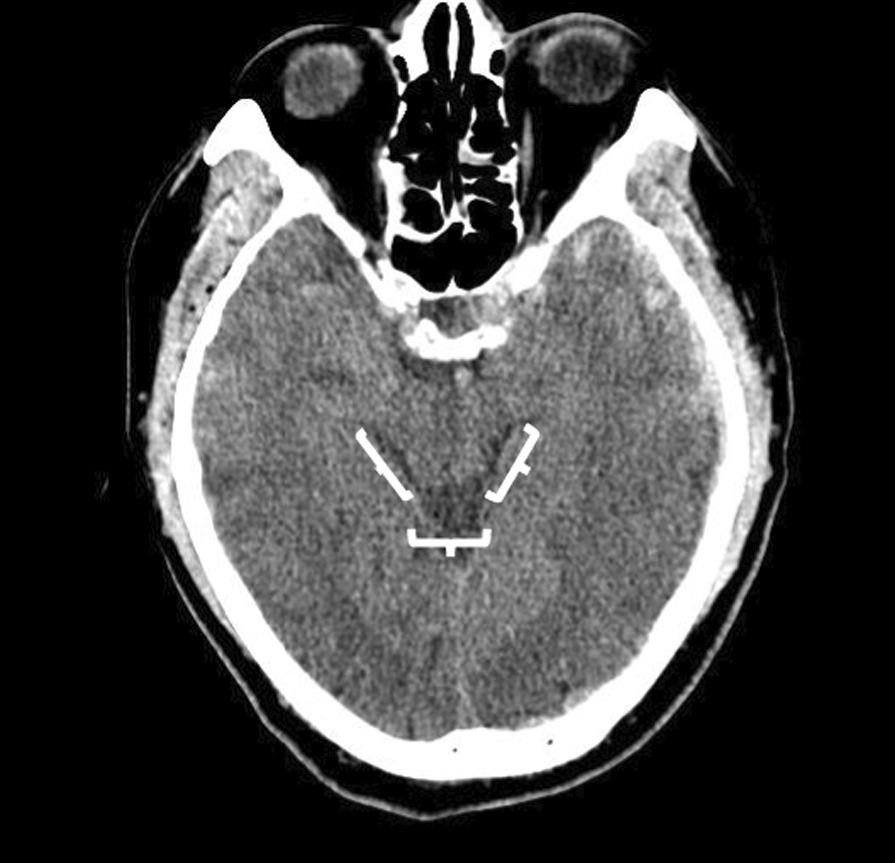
Evaluation of the basal cisterns on computed tomographic scan
